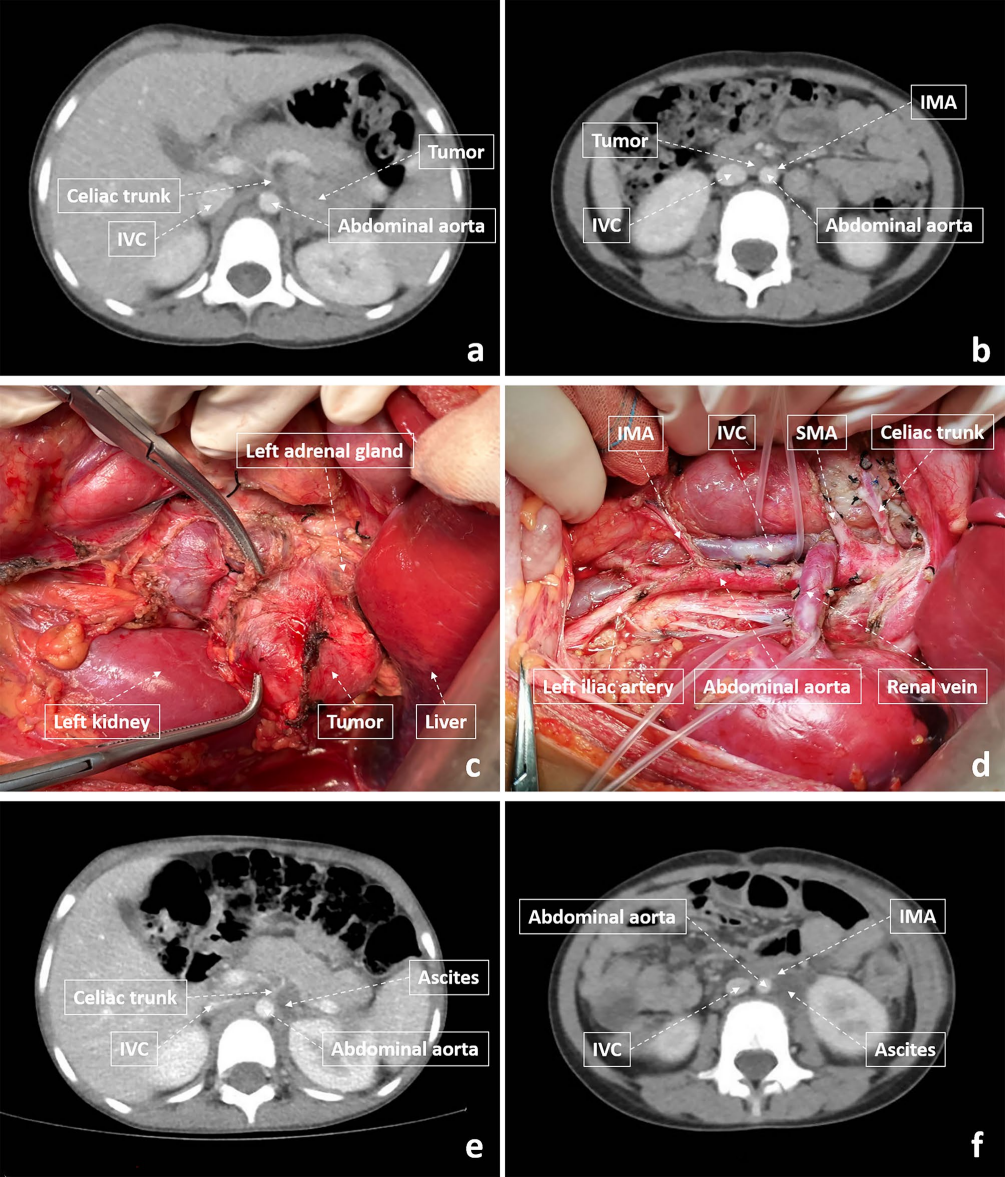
The preoperative, operative and postoperative images of NB. (a-b) Preoperative CT scan showed that the tumor wrapped around retroperitoneal vessels. (c-d) Operative photos before, and after adrenalectomy with lymphatic dissection. (e-f) The CT scan of one month after operation.
